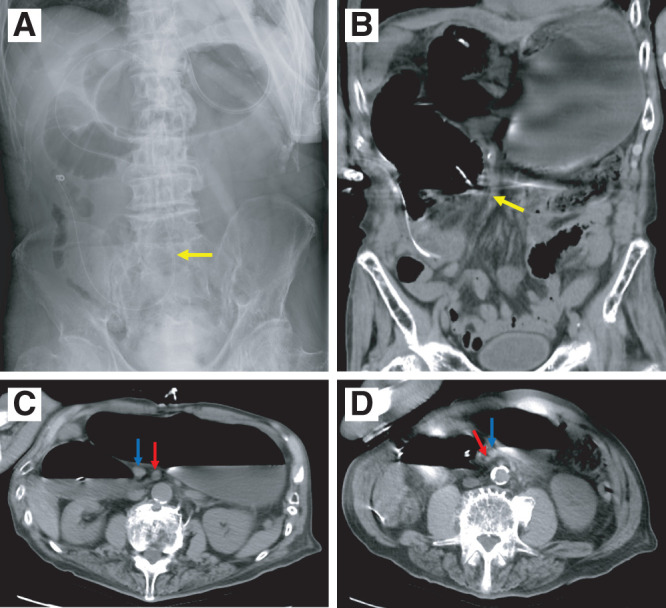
Imaging findings in small bowel volvulus. Anteroposterior abdominal X-ray (A) and abdominal multidetector CT images—coronal section (B), and axial sections at the L2 vertebral level (C) and L4 vertebral level (D). A percutaneous endoscopic gastrojejunostomy tube is in place. The third portion of the duodenum (yellow arrow) coursed caudally without passing between the AA and the SMA. At the L2 vertebral level, the SMV (blue arrow) was located to the right of the SMA (red arrow), whereas at the L4 vertebral level, the SMV was located to the left of the SMA. AA, abdominal aorta; SMA, superior mesenteric artery; SMV, superior mesenteric vein
